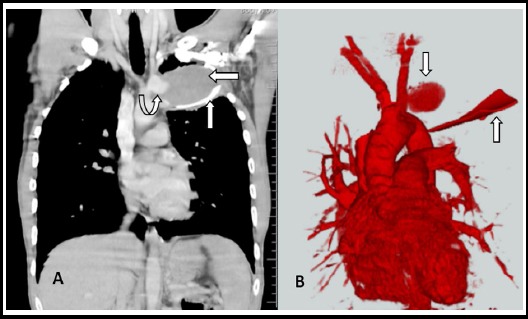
(A+B): MDCT of Chest (A) shows a saccular aneurysm of mediastinal part of left common carotid artery (curved up arrow) surrounded by thrombosis (left arrow) with a cylindrical linear metallic foreign body (up arrow) and CT Angiography. (B) confirmed a saccular aneurysm of left proximal common carotid artery (down arrow) and a cylindrical linear metallic foreign body (up arrow).
